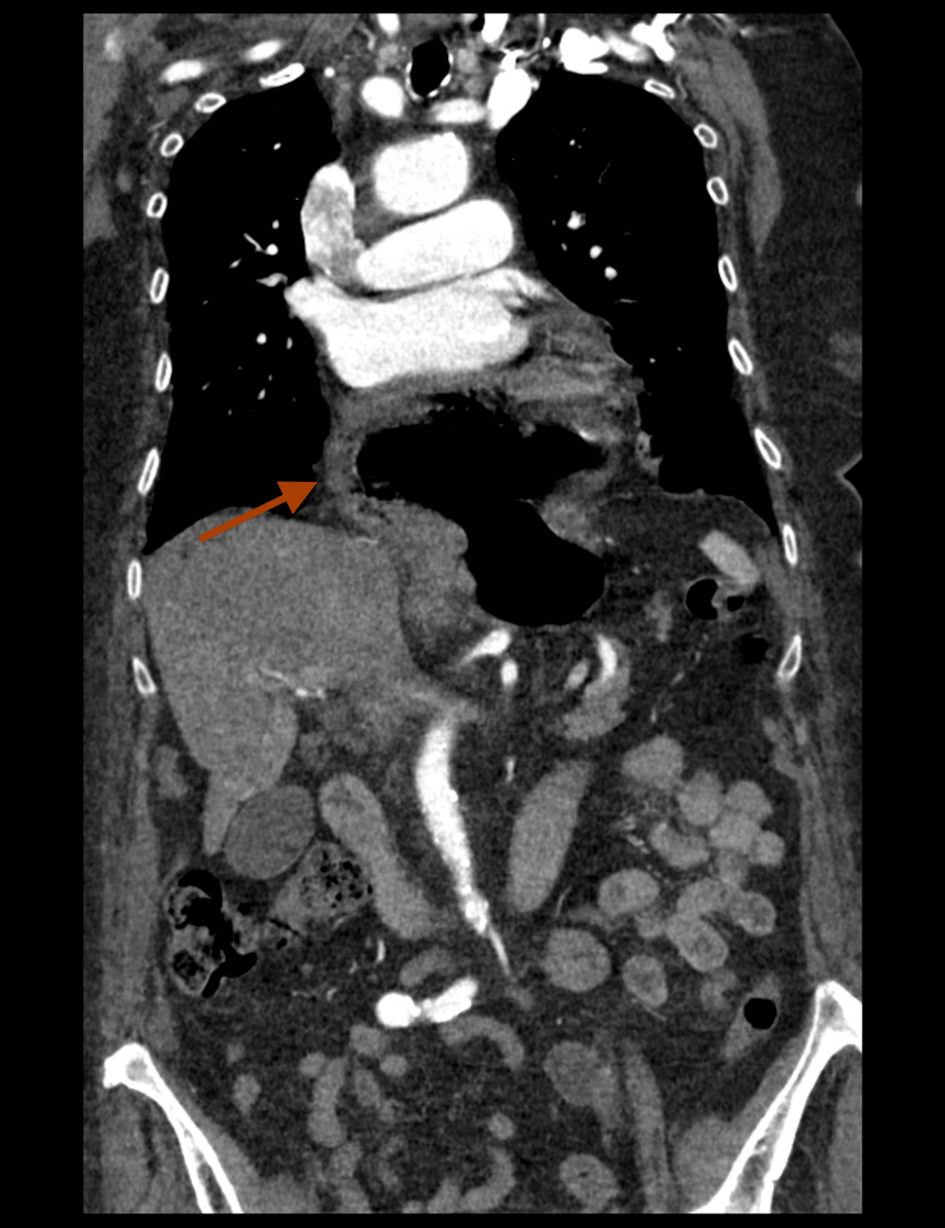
Chest CT showing incarcerated sliding hiatal hernia (coronal). The red arrow points to the displacement of the gastric fundus into the thoracic cavity above the diaphragm. The hernia compresses the heart and lower lung fields.
